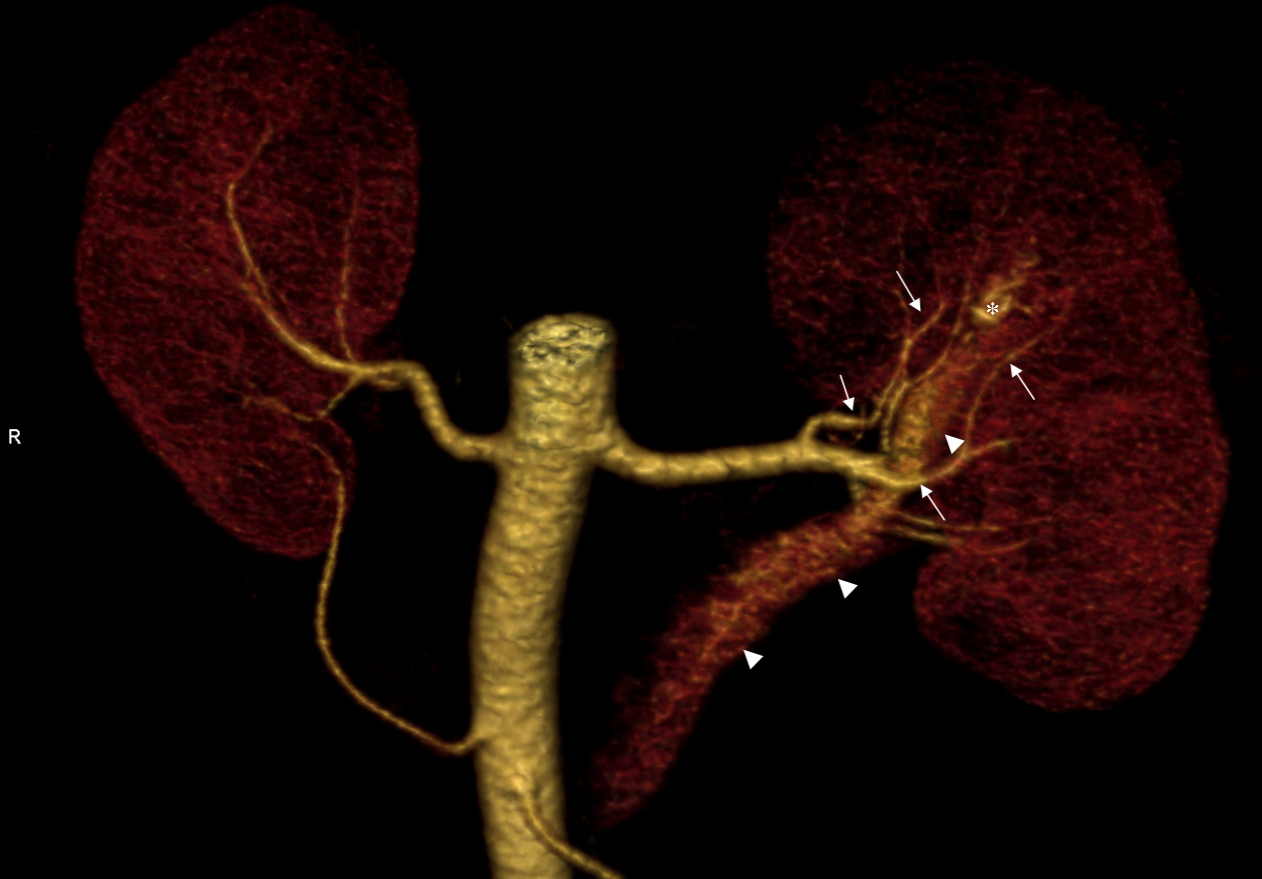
Computed tomography with iodinated contrast enhancement shows the presence of tortuous blood arterial vessel with thin arterial ramifications of spiral form. The arrowhead indicates the renal vein, the arrows indicate the renal artery, and the asterisk indicates the renal AVM.
